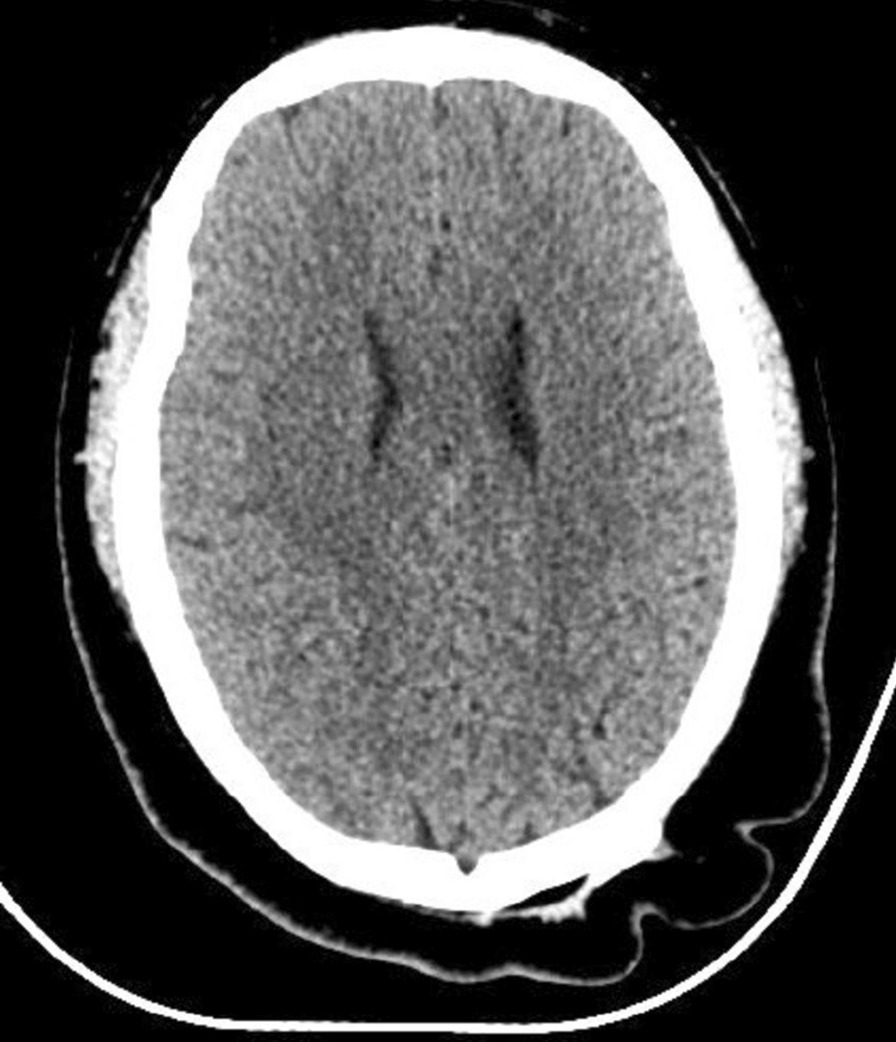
CT scan images revealed a thickened dermis and excessive growth of the scalp, forming the characteristic scalp folds, without abnormalities of bone or intracranial tissue
